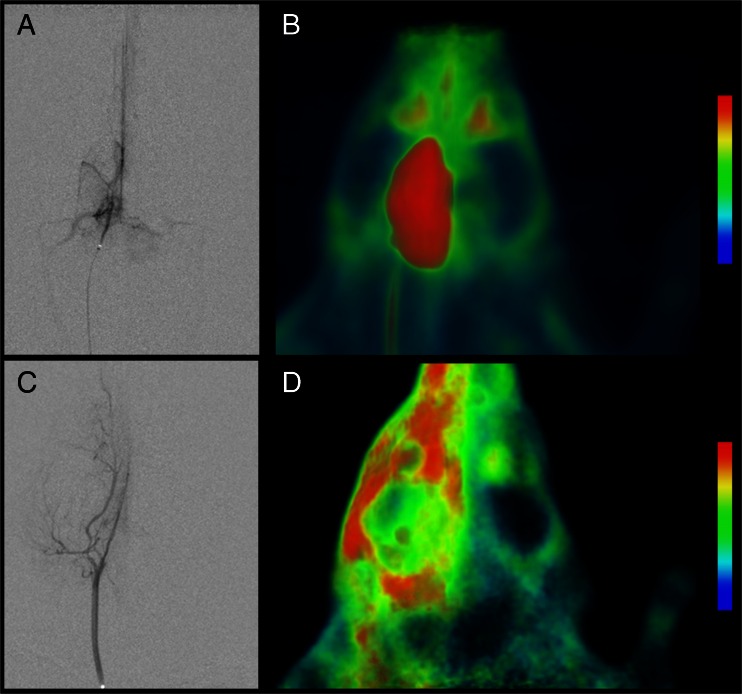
Distribution of ^18^F-FDG and iodine contrast media following selective intra-arterial injections in the internal and common carotid arteries. a Digital subtraction angiography (*DSA*) and b
